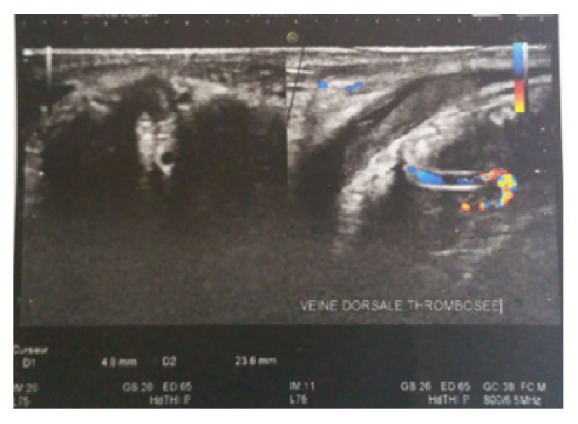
Doppler coupled with USS showing an intraluminal thrombosis (weight arrow) of the dorsal vein of the penis.
